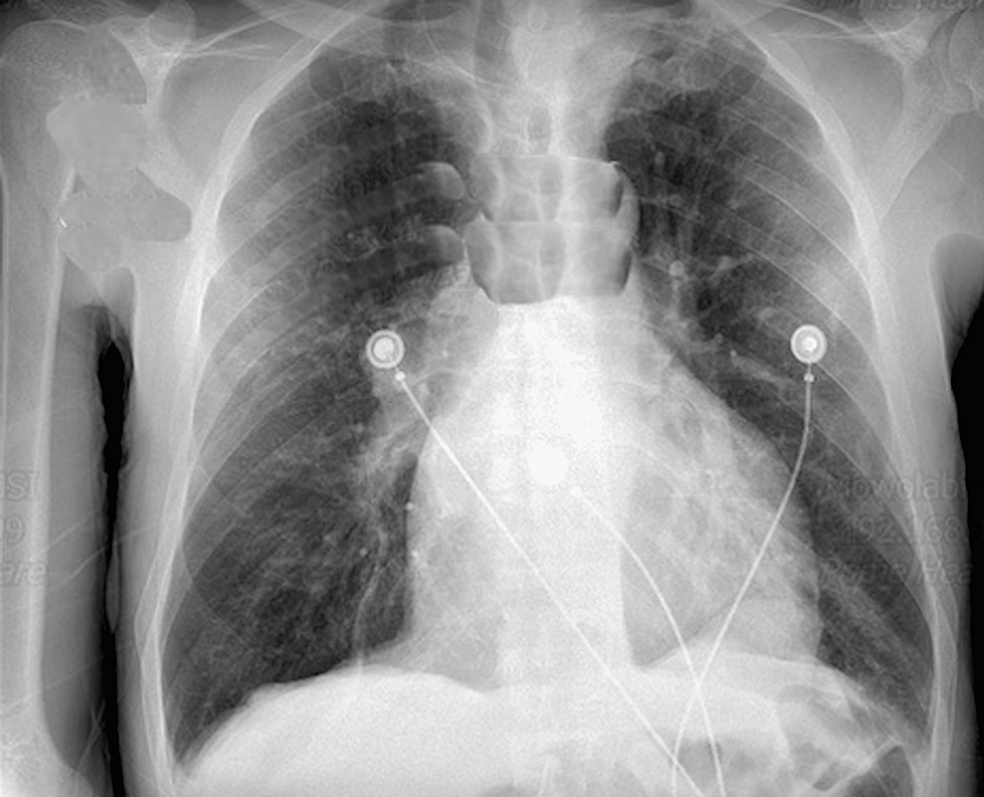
Chest X-ray showing no acute pathology.
